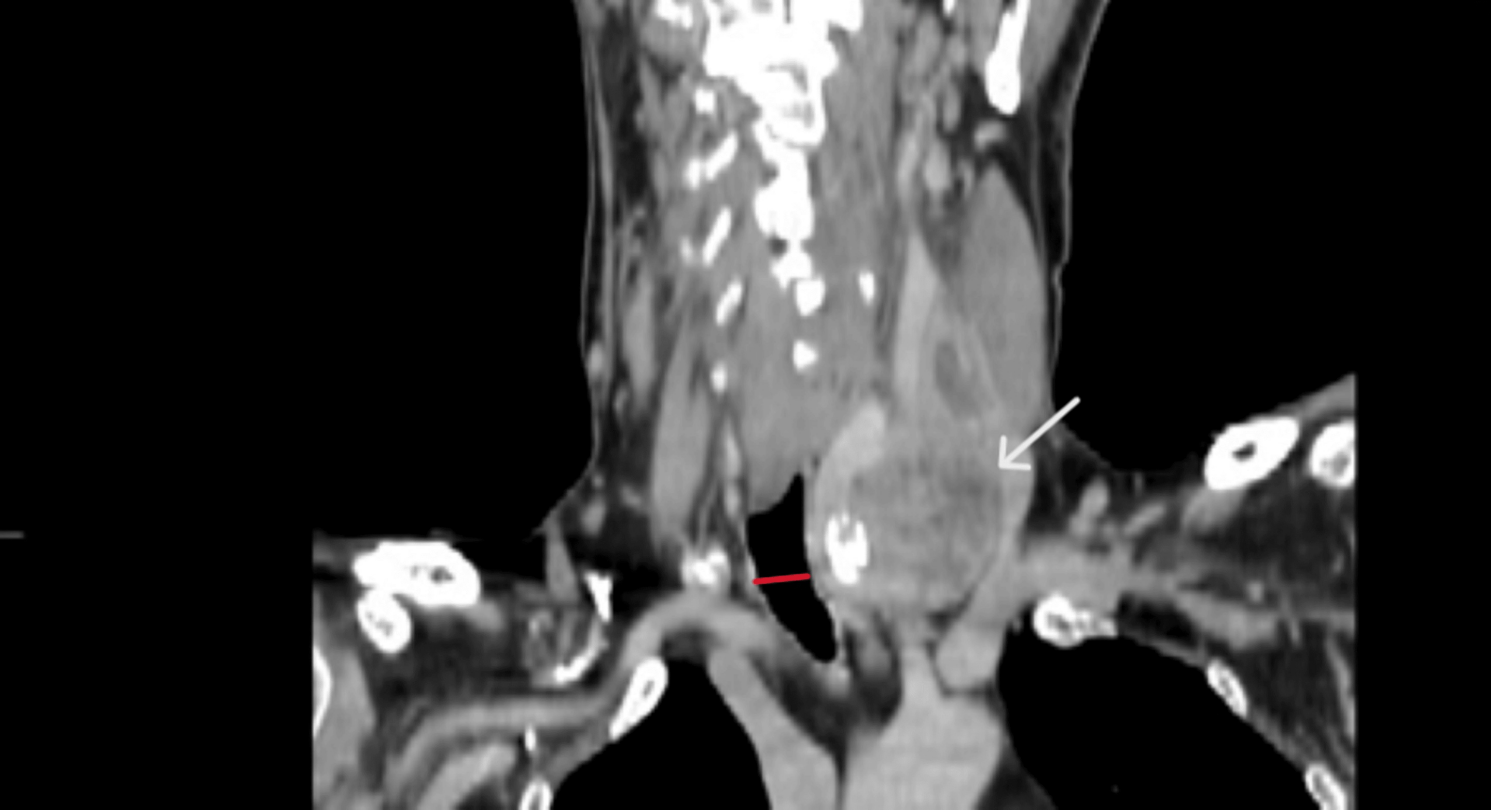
CT - coronal. Tumor with cystic element (white arrow) and calcification, compressing the carotid artery and jugular vein. The narrowed tracheal segment is 1.27 cm in diameter (red line).
